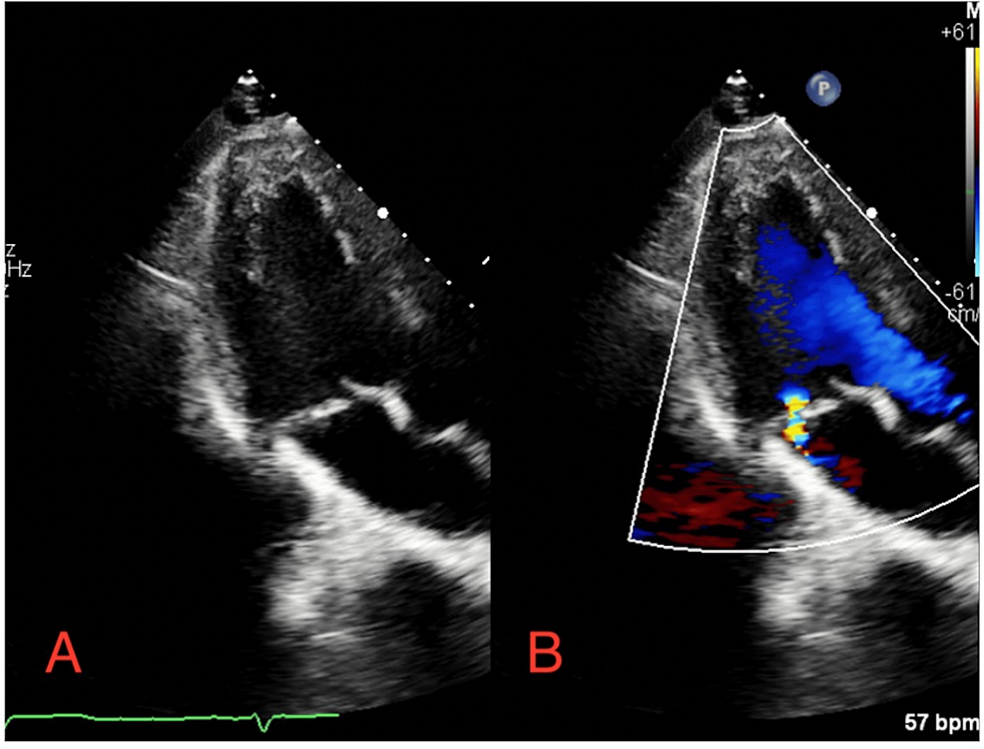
(A) Transthoracic echocardiogram in apical view showing notable MVP with a (B) posteriorly directed jet of mild mitral valve insufficiency with Doppler MVP: mitral valve prolapse
